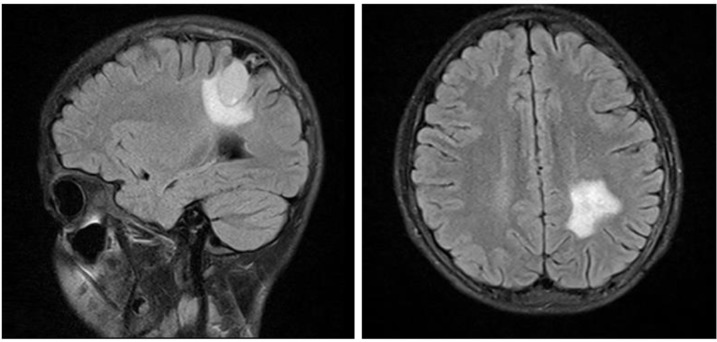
Follow-up brain MRI after one year showing surgery scar and no recurrence (original images)
